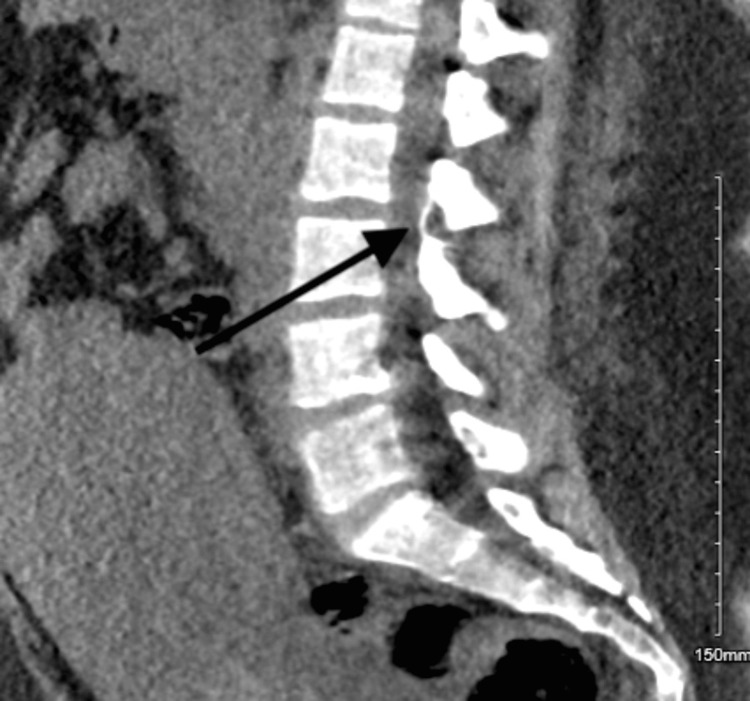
CT scan of the spine at the lumbar (spinal canal). The sequence of the CT imaging indicates the entry and the end of the epidural catheter. It can be noted it enters the spinal canal and goes upward within the posterior aspect of the spinal canal, spanning 1.7 cm.
